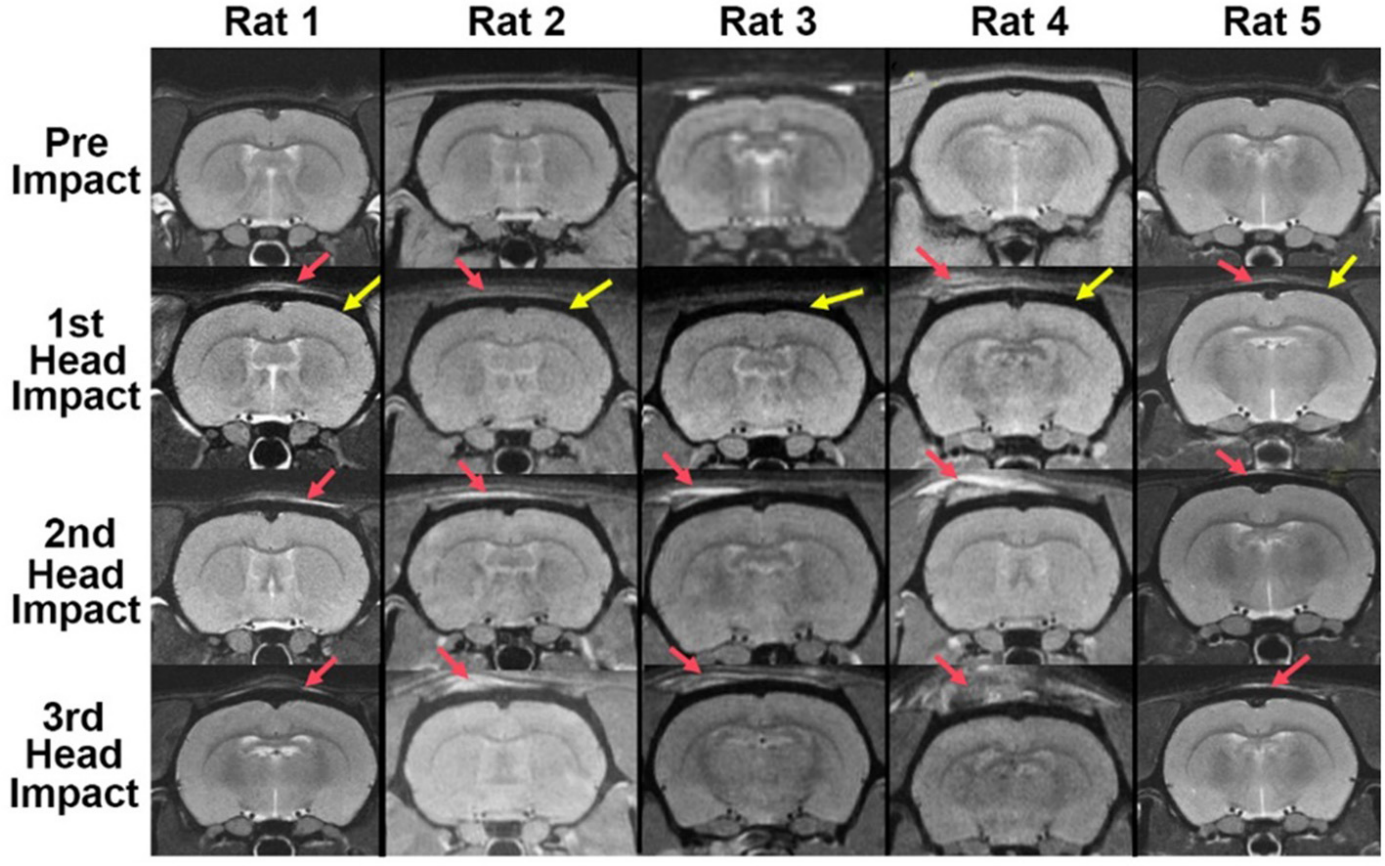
Brain neuroanatomy following repetitive mild head impacts. Mild head impact without evidence of brain damage. Shown are axial sections of T2-weighted anatomical images taken within 1 h of mild head impact. The site of impact noted by the red arrow can be identified by the high intensity signal reflecting the edema on the tissue. The yellow arrows point to the black contour of the cranium. There is no visible evidence of structural brain damage.
